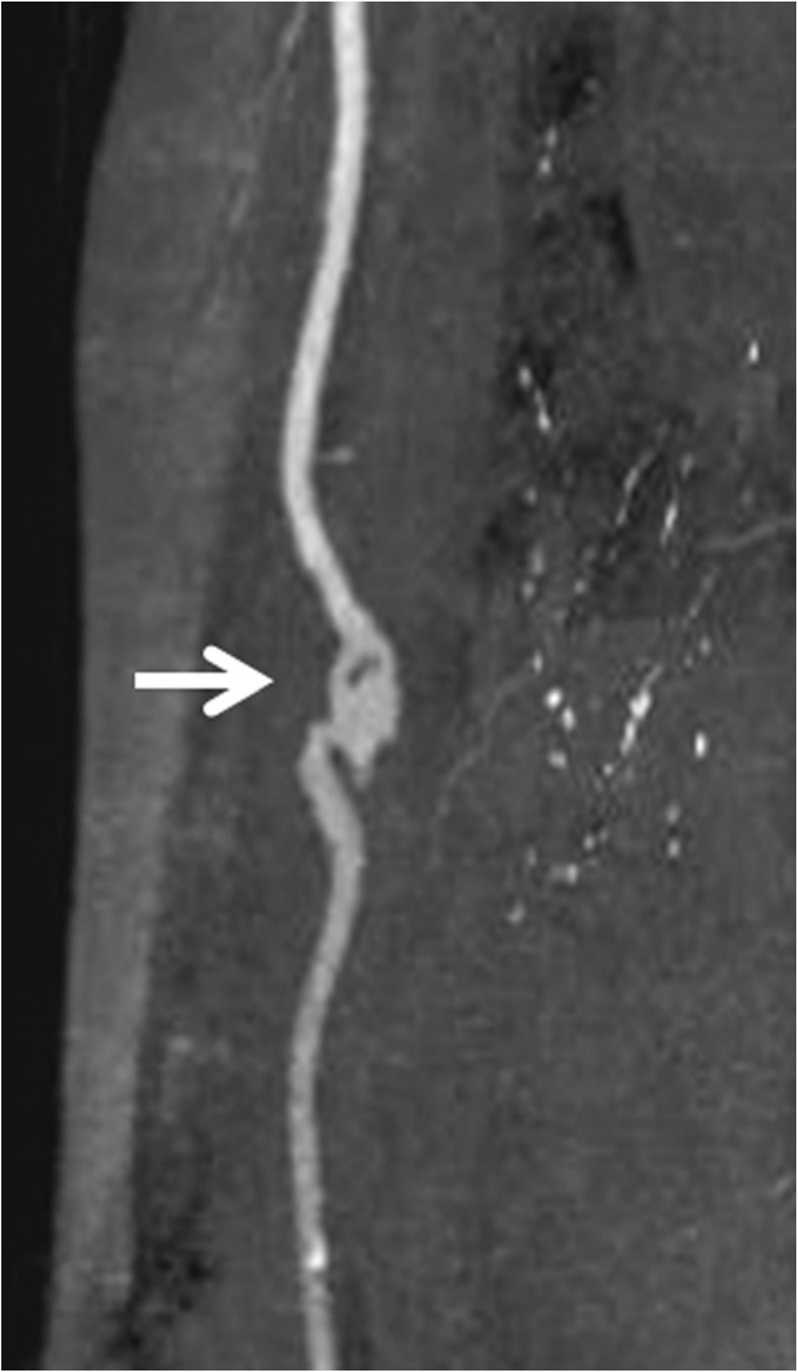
Right brachial artery (three-dimensional computed tomography [3D-CT]). This was believed to be an infected aneurysm associated with the dialysis procedure; the aneurysm was present on the central side of the dialysis puncture site. It was believed that this site was infected because the fever was initially limited to the day of dialysis.
